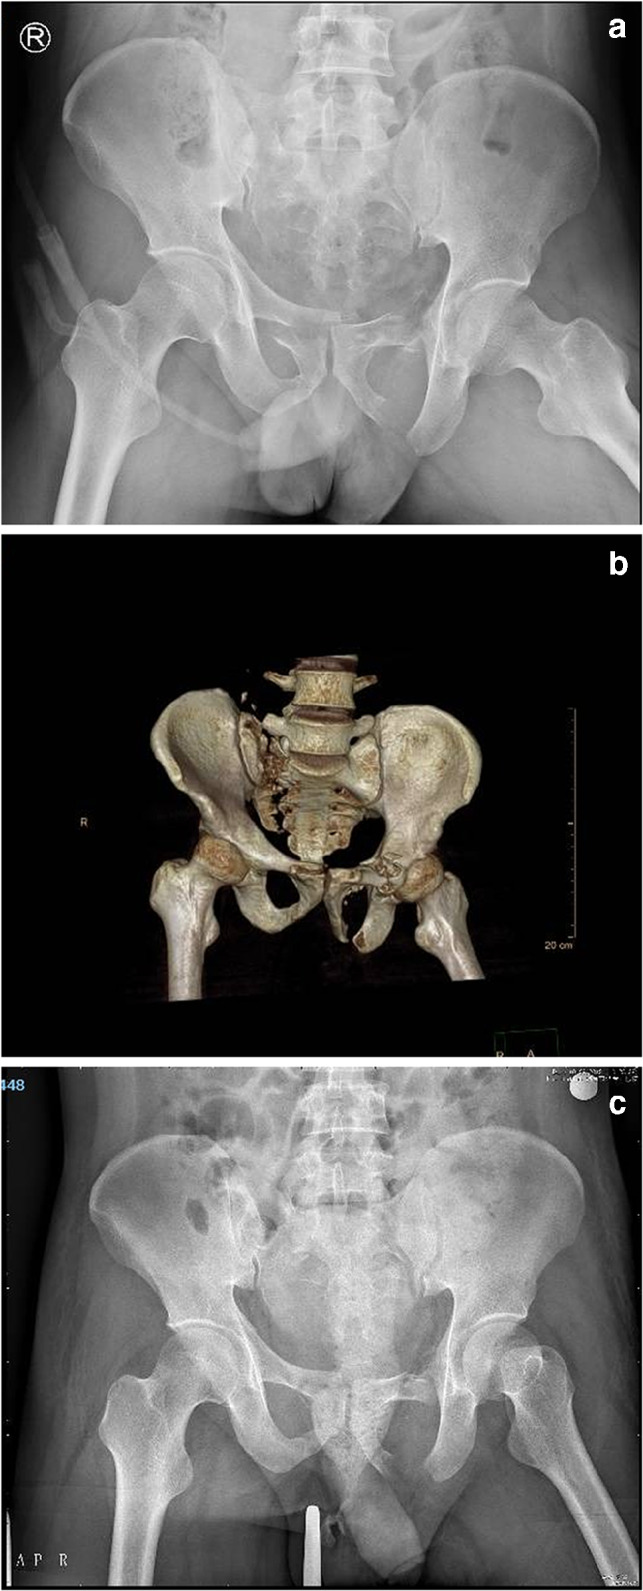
Example images from the cases of unstable pelvic fractures. a X-ray image of a male, 46 years old, with crush injury showing vertical displacement of the right sacroiliac joint, bilateral pubic ramus fracture, tile type C1. b 3D computed tomography (CT) reconstruction of a right sacral alar fracture, vertical displacement of the right sacroiliac joint and bilateral ramus pubis fracture. c Right lower limb bone traction, X-ray shows: right sacroiliac joint vertical displacement correction reduction, pelvic anterior and posterior ring reduction
